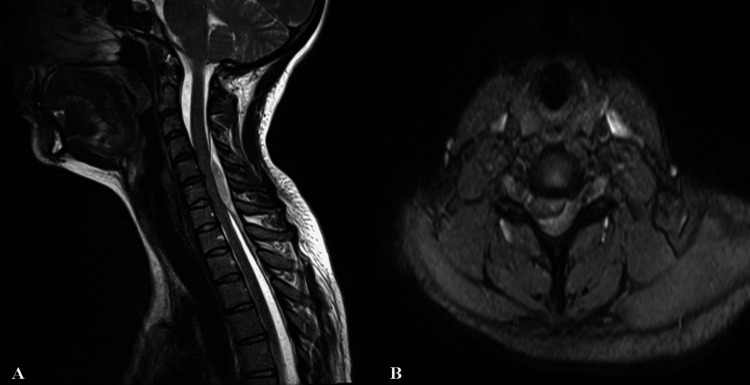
Preoperative cervical sagittal (A) and axial (B) MR images of the patient revealing an epidural hematoma exerting a compressive effect from the right posterolateral aspect
